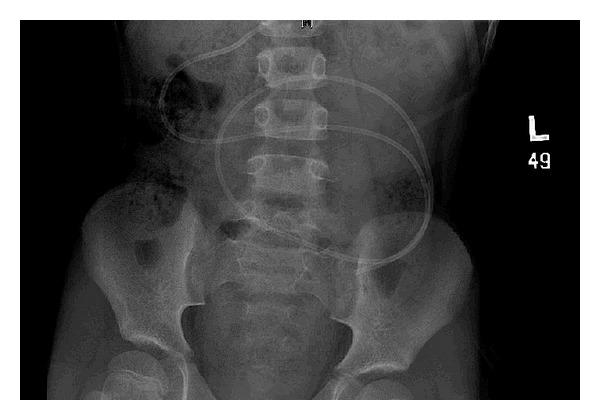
Abdominal X-ray showing the peritoneal catheter with early large knot formation.
